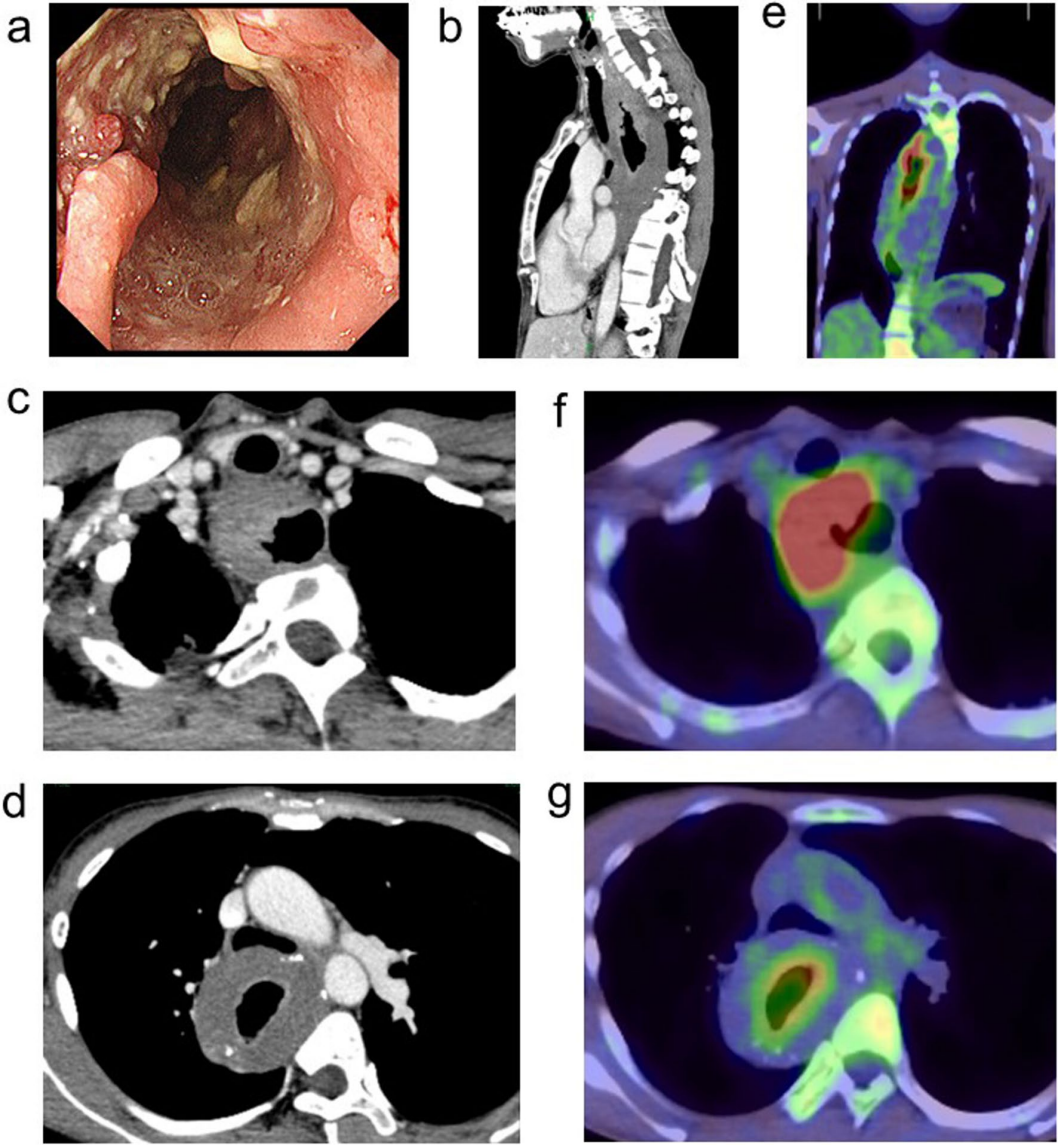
Findings before treatment. a Esophagoscopy shows a circumferential ulcerated lesion and stenosis 15–29 cm from the incisors. b–d Contrast-enhanced computed tomography (CT) image: abnormal circumferential wall thickening in parts of the cervical and most of the thoracic esophagus. Cervical lesion invading the adventitia (cT3).  e–g Positron emission tomography (PET)-CT images show uptake in the cervical and upper esophagus and no uptake in the muscular layer of the middle and lower esophagus
